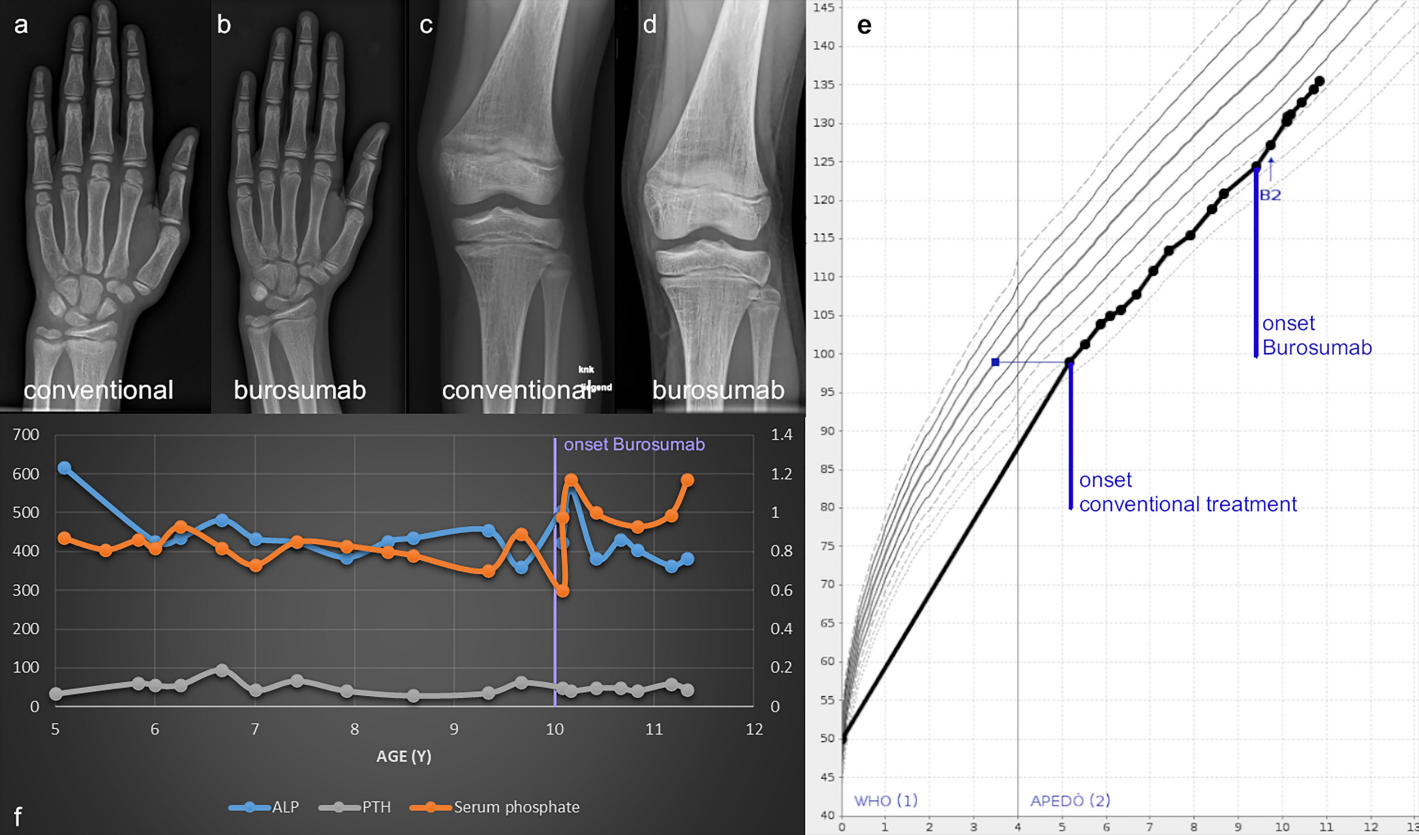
Wrist and knee radiographs obtained before therapy onset (A, C) and at the one-year follow-up show resolution of rickets (B, D) in case 2. Linear growth, onset of treatment and thelarche (B2) are marked on the Austrian reference growth chart for girls (E). The course of relevant biochemical parameters shows increase of serum Phosphate and normalization of ALP with onset of Burosumab treatment around 10 years of age (F).
